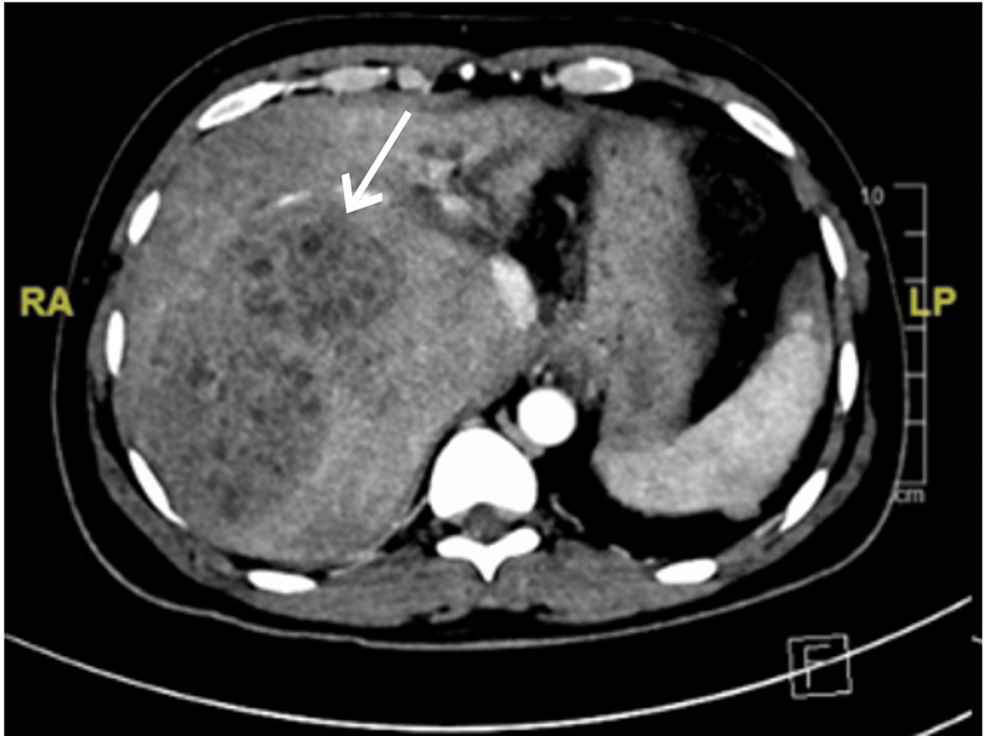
Axial computed tomography triphasic scan showing an enlarged liver (measuring 21 cm). A multiloculated complex cystic lesion was observed, representing an abscess measuring 9.7 x 6.7 x 10 cm in the anteroposterior, transverse, and craniocaudal dimensions occupying the right liver lobe, segments VII and VIII.
